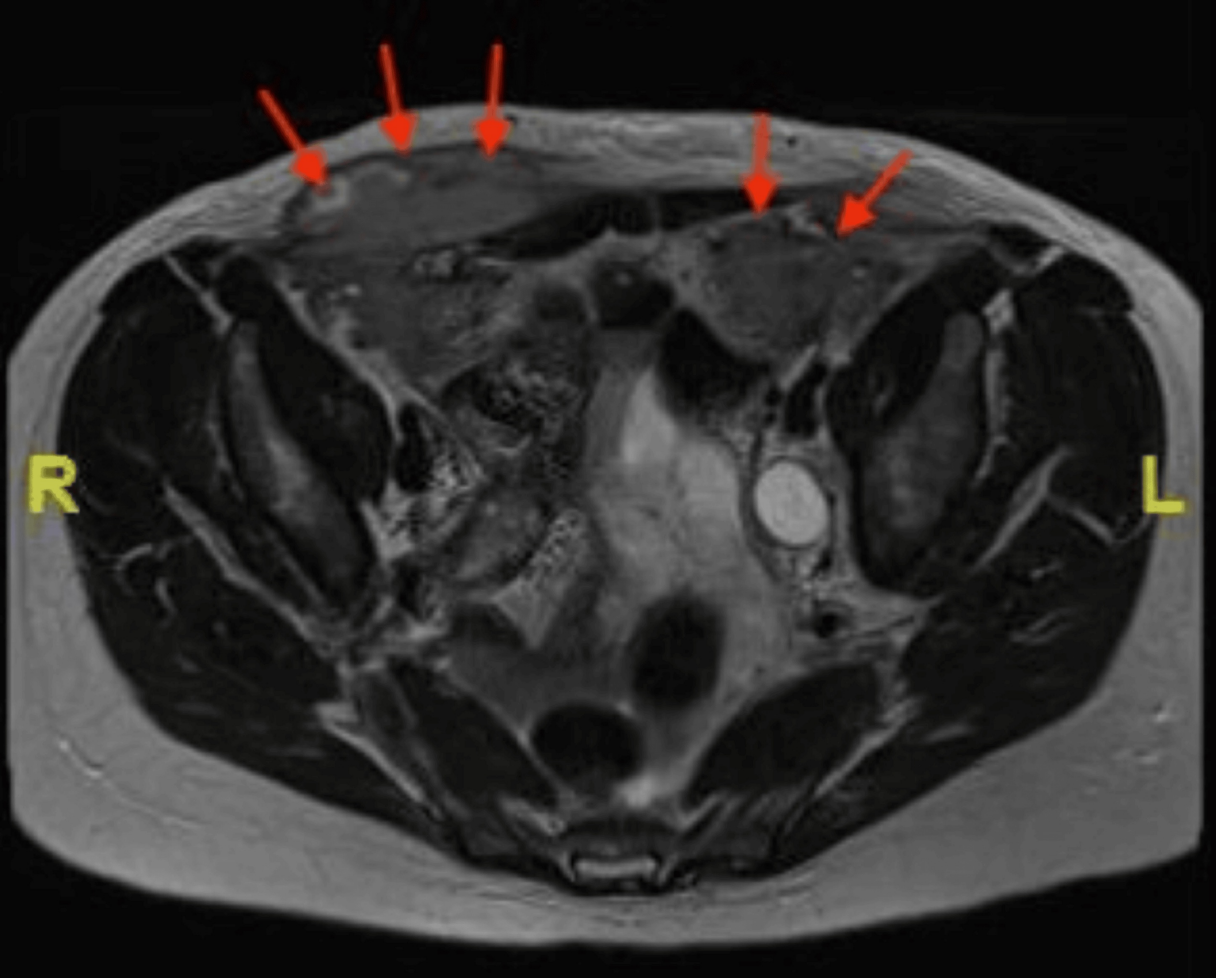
Axial MRI pelvis showing solid lesions within both iliac fossas.
